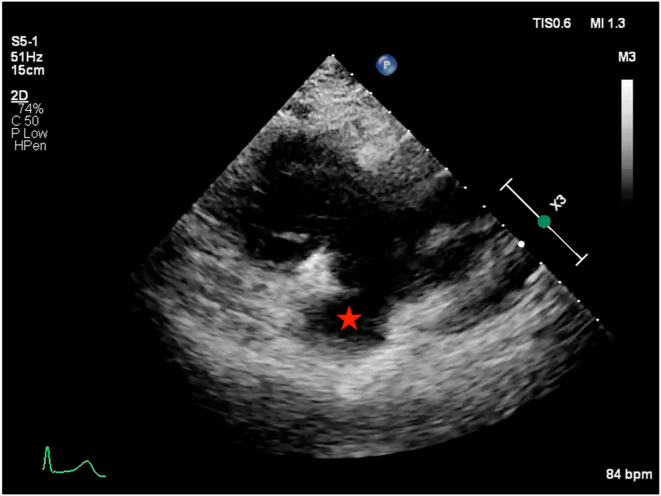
Two-dimensional TTE, parasternal right ventricular inflow view, demonstrates a dilated right heart with dilated CS *(star)*.
